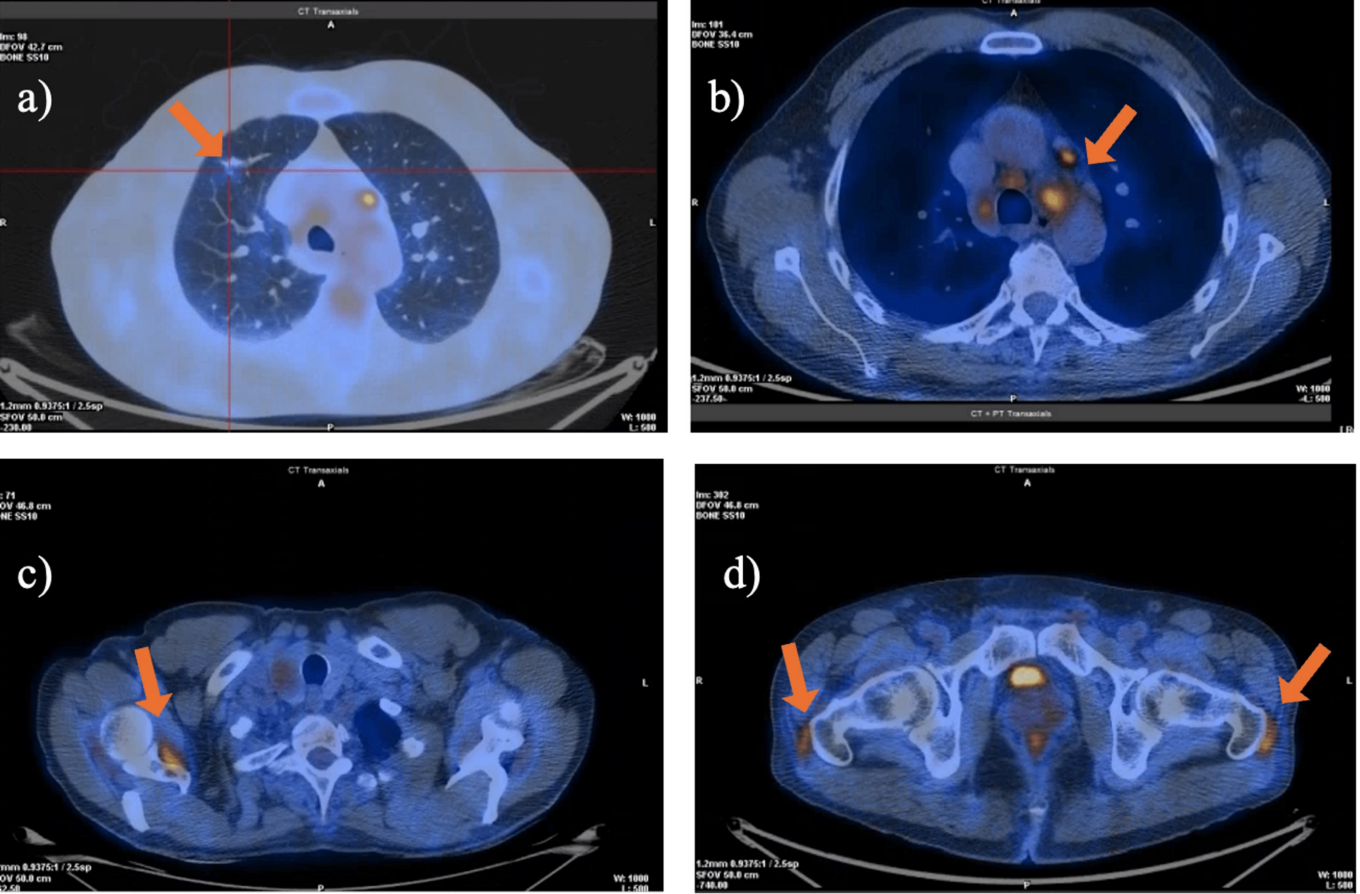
Whole-body 18F-fluorodeoxyglucose positron emission tomography-computed tomography (18F-FDG PET-CT). (a) Moderate FDG uptake (qSUVmax = 1.93) in a small right upper-lobe pulmonary density (arrow). (b) Hypermetabolic mediastinal and hilar lymph nodes involving the bilateral paratracheal, para-aortic, subaortic, subcarinal, and hilar stations (qSUVmax = 5.01 on the right) (arrow). (c) Symmetric FDG uptake in the bilateral glenohumeral and sternoclavicular joints and along the inferior scapular margins (arrow). (d) Increased FDG uptake over the bilateral femoral greater trochanters (arrows). qSUVmax: quantitative maximum standardized uptake value
